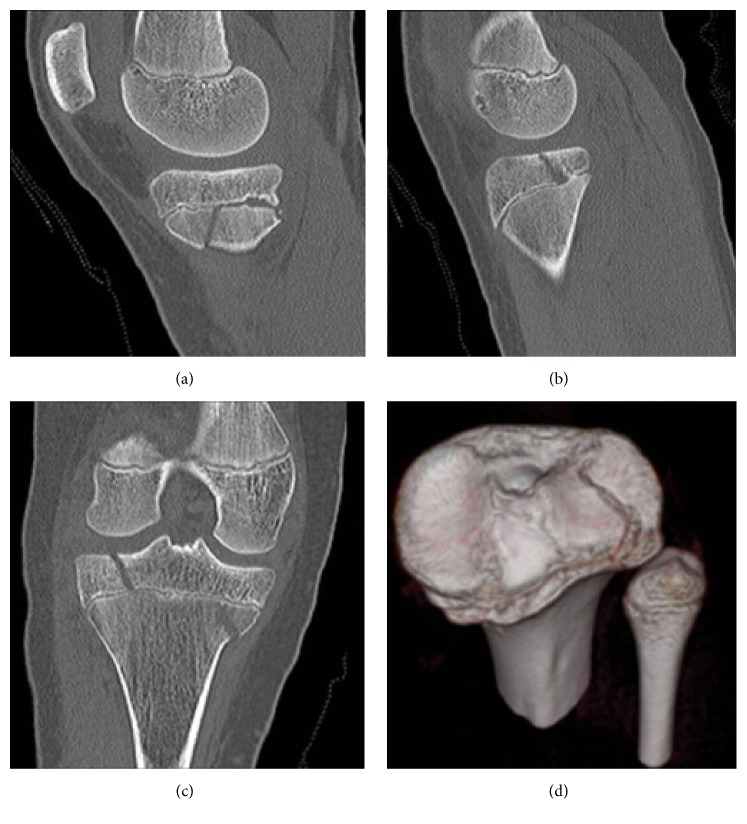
Sagittal, coronal, and 3D reformatted CT images of the right knee demonstrating the coronal fracture medially in the metaphysis (a), laterally in the epiphysis (b), sagittal fracture lines (c), and the rotational component in the axial plane (d).
